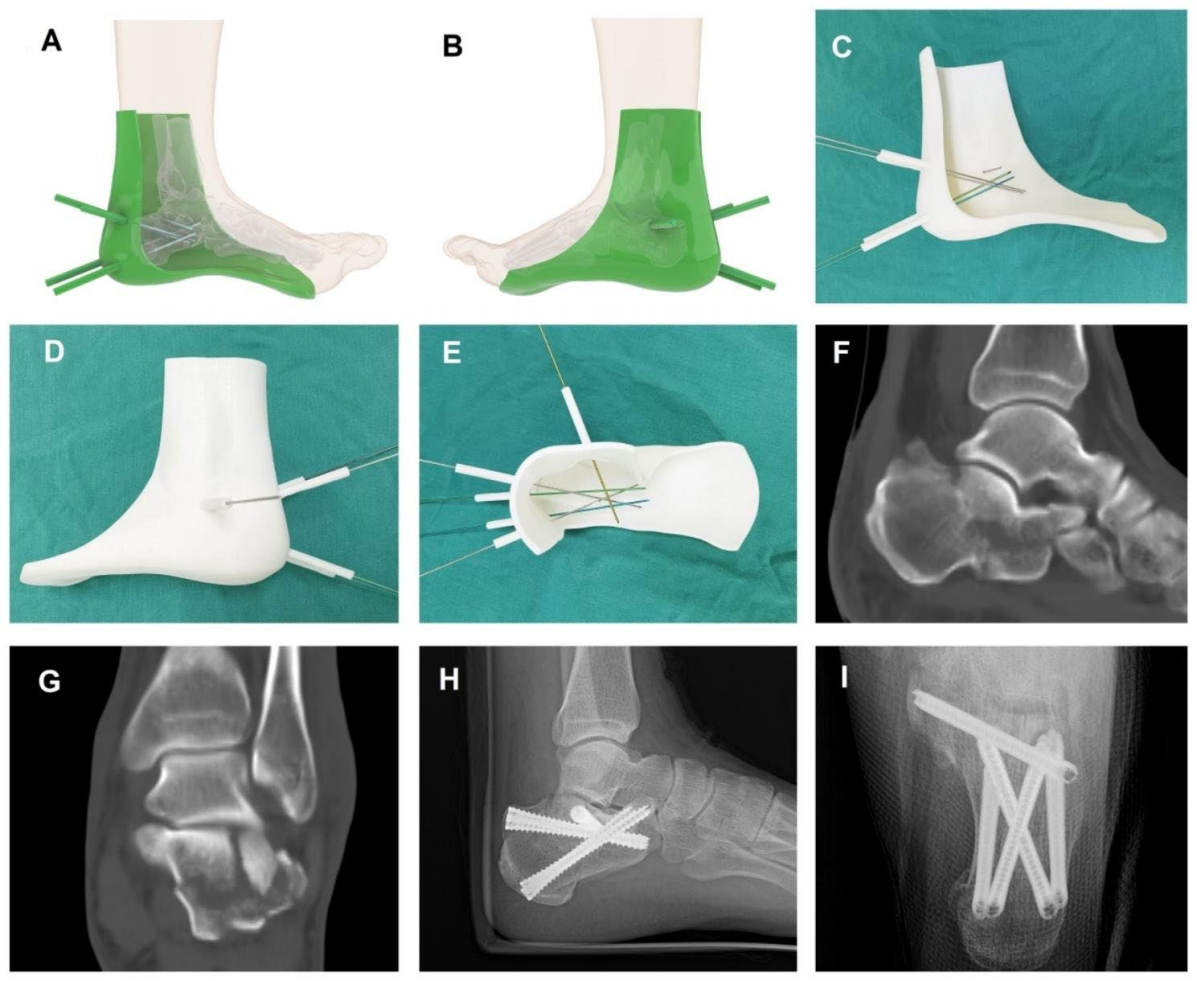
The design, fabrication, and application of 3D printed cast. A,B The reconstruction and design of 3D model based on CT data. C, D, E The 3D printed cast with five cannulas for insertion of 1.5 mm K-wires. F, G CT images of a patient (male, 42 years of age) with displaced intra-articular calcaneal fracture, showing sustentaculum tali nondisplaced. H, I A fractured calcaneus treated with percutaneous reduction and 3D printed cast assisted screw fixation
